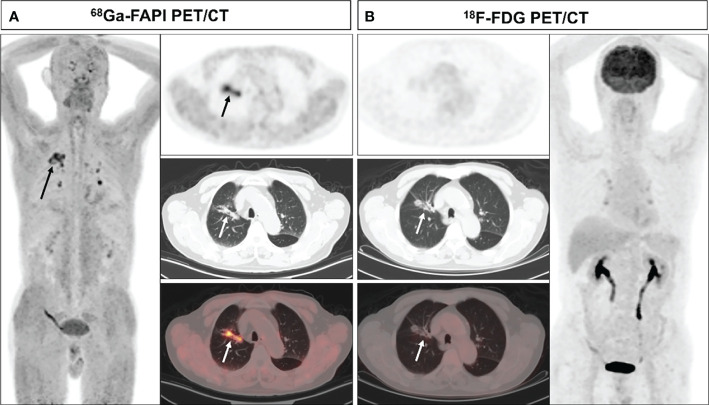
A 70-year-old male (patient 9) diagnosed with adenocarcinoma. ^68^Ga-FAPI PET/CT (A) revealed an adenocarcinoma lesion with increased FAPI uptake (solid arrows, SUVmax=6.3), while ^18^F-FDG PET/CT did not reveal any significant uptake in the primary lesion (B) solid arrows).
